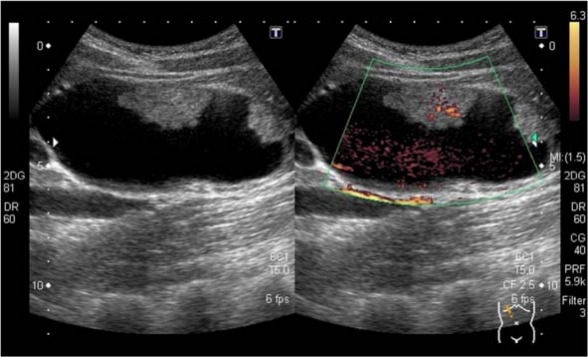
Abdominal ultrasonography showed two tumors extending into the common bile duct. Doppler ultrasound showed a blood flow signal within the tumor
